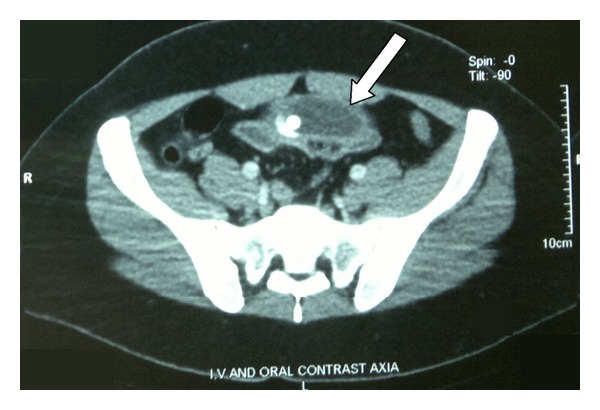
Abdominal CT scan showing the deflated intragastric balloon migrated to the small bowel (white arrow).
